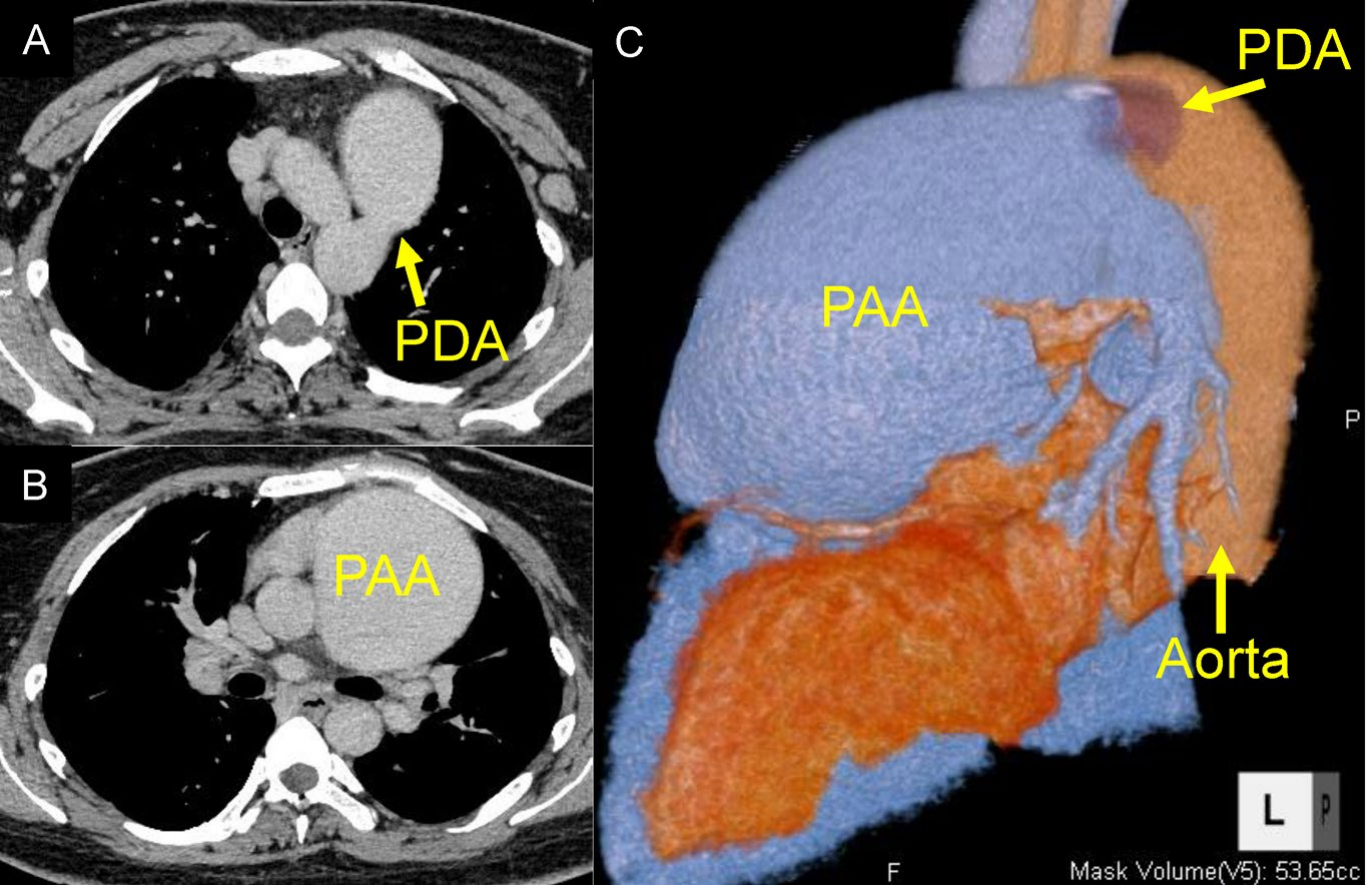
Preoperative chest computed tomography (CT) and three-dimensional reconstructed CT. (A) Chest CT showing a patent ductus arteriosus (PDA) with an internal diameter of 15 mm. (B) Chest CT revealing a giant pulmonary artery aneurysm (PAA) measuring up to 8 cm in the pulmonary artery trunk. (C) Three-dimensional reconstructed CT highlighting the large PAA and PDA in relation to the aorta
